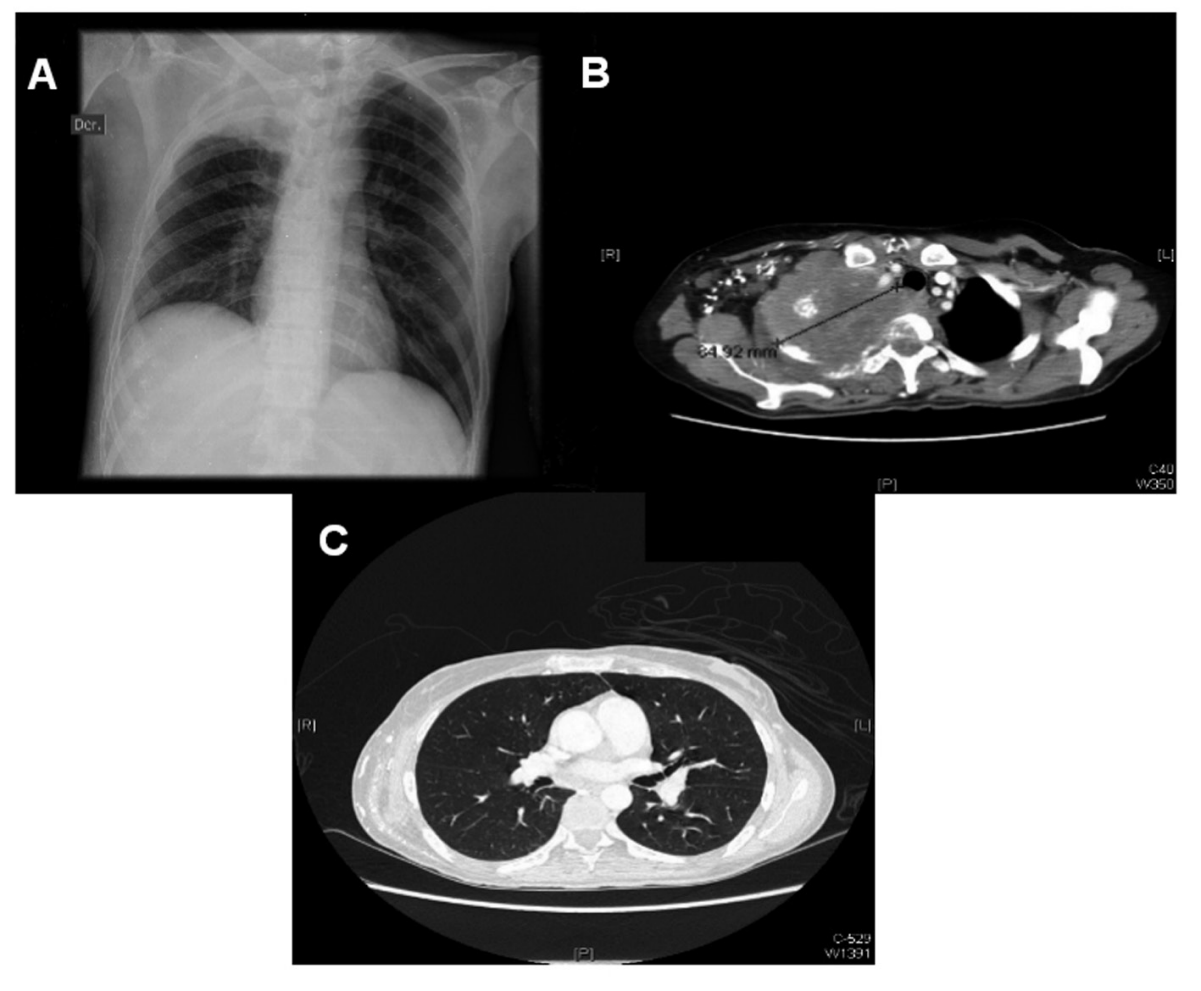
Chest x-ray showing right apical opacity (A). Chest CT showing large apical lung mass, invading vertebral body (B). Rest of lung parenchima is intact, there is no hilar adenopathy (C).
